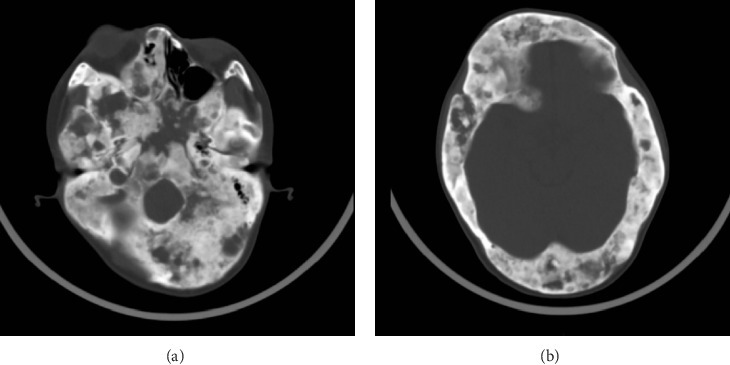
Cranial CT revealed: (A) bony lesions in the skull base and (B) bony lesions in the calvarium.
